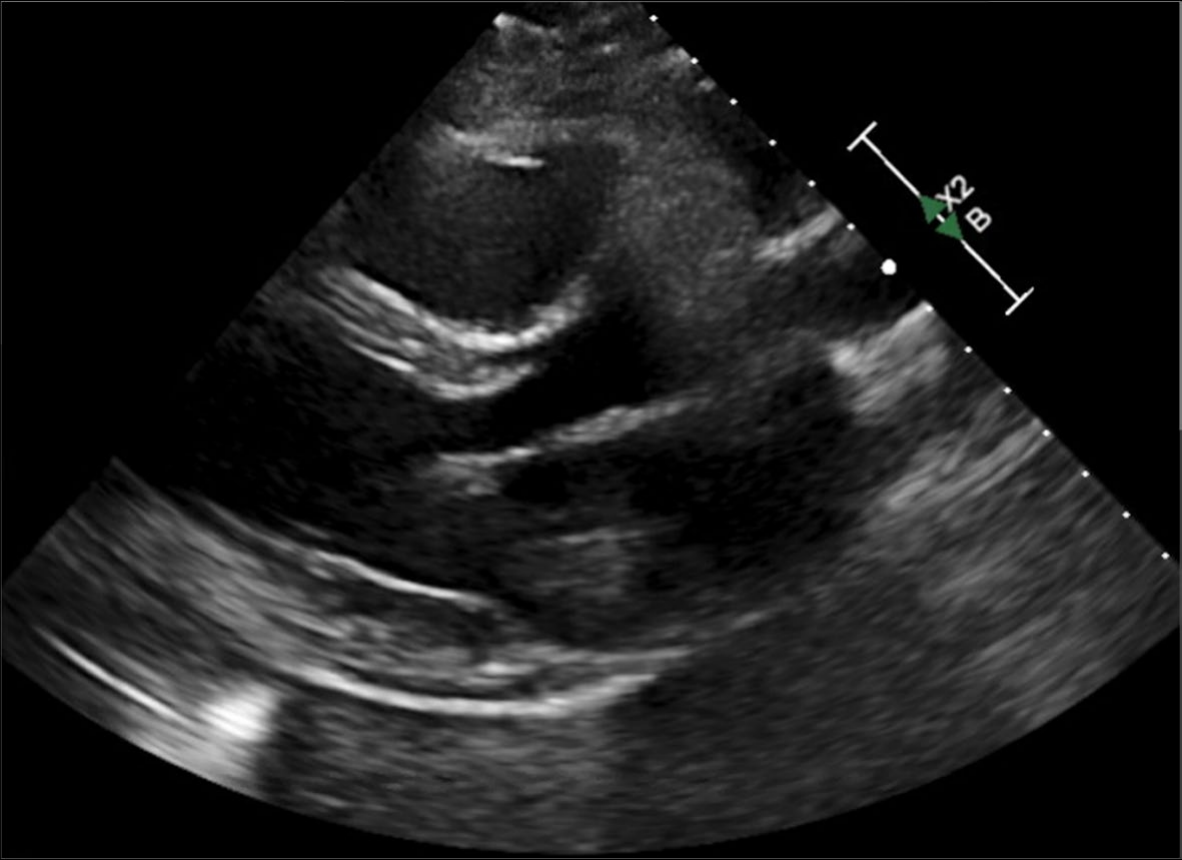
Echocardiogram showing trivial pericardial effusion
